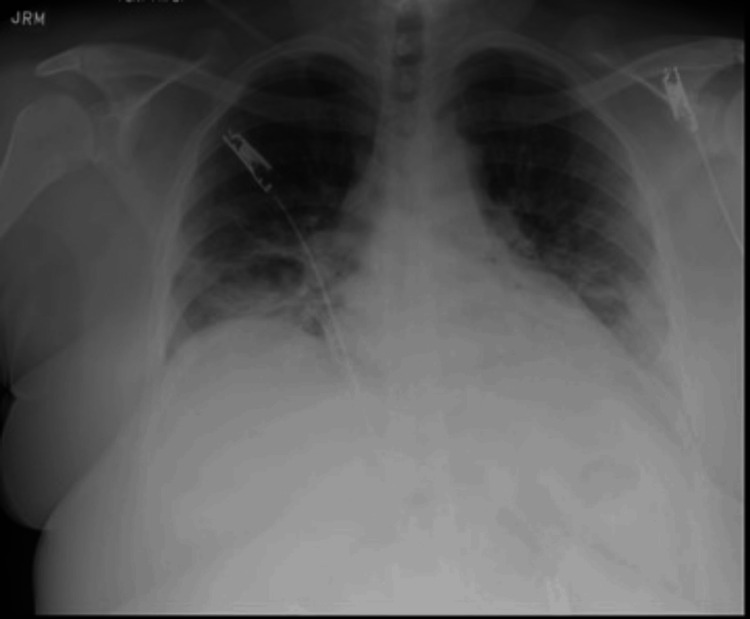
A chest X-ray taken 10 days after the initial presentation revealed residual basilar airspace opacity, as compared to Figure 2
